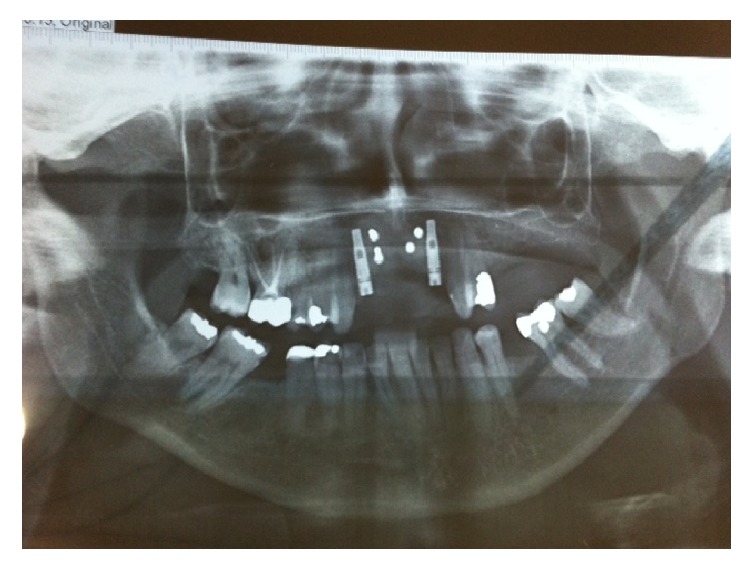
Panoramic radiograph after 10 months postoperatively.
